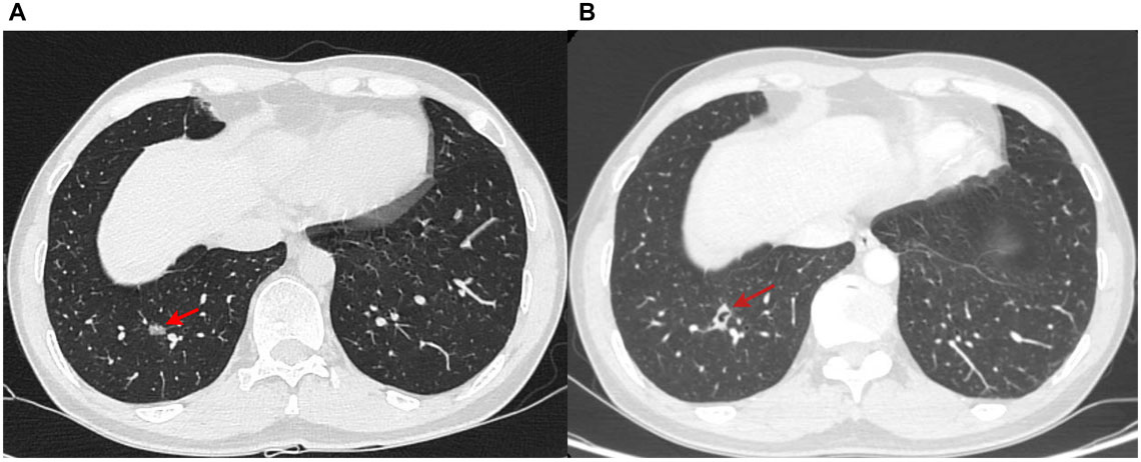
(A) Computed tomography image before ablation. (B) Computed tomography image of 2 months after ablation.
